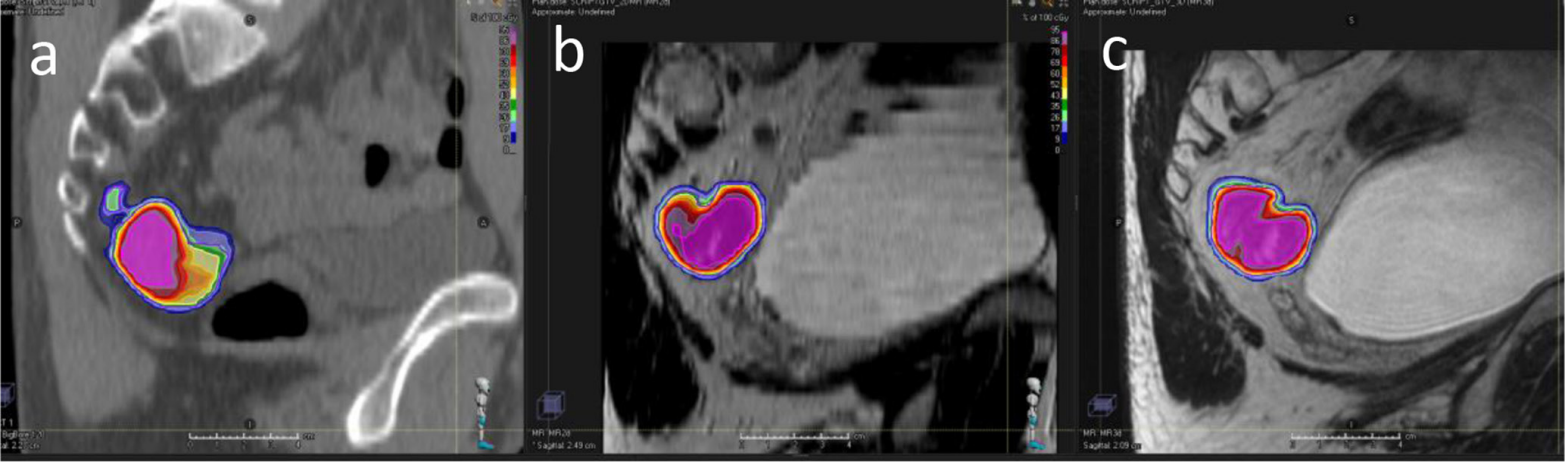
Consensus agreement map for GTVp. (a) CT, (b) 2D-MRI and (c) 3D-MRI. Pink represents 95% agreement between observers in the volume to be contoured in the delineated structure. GTVp, gross tumour volume.
